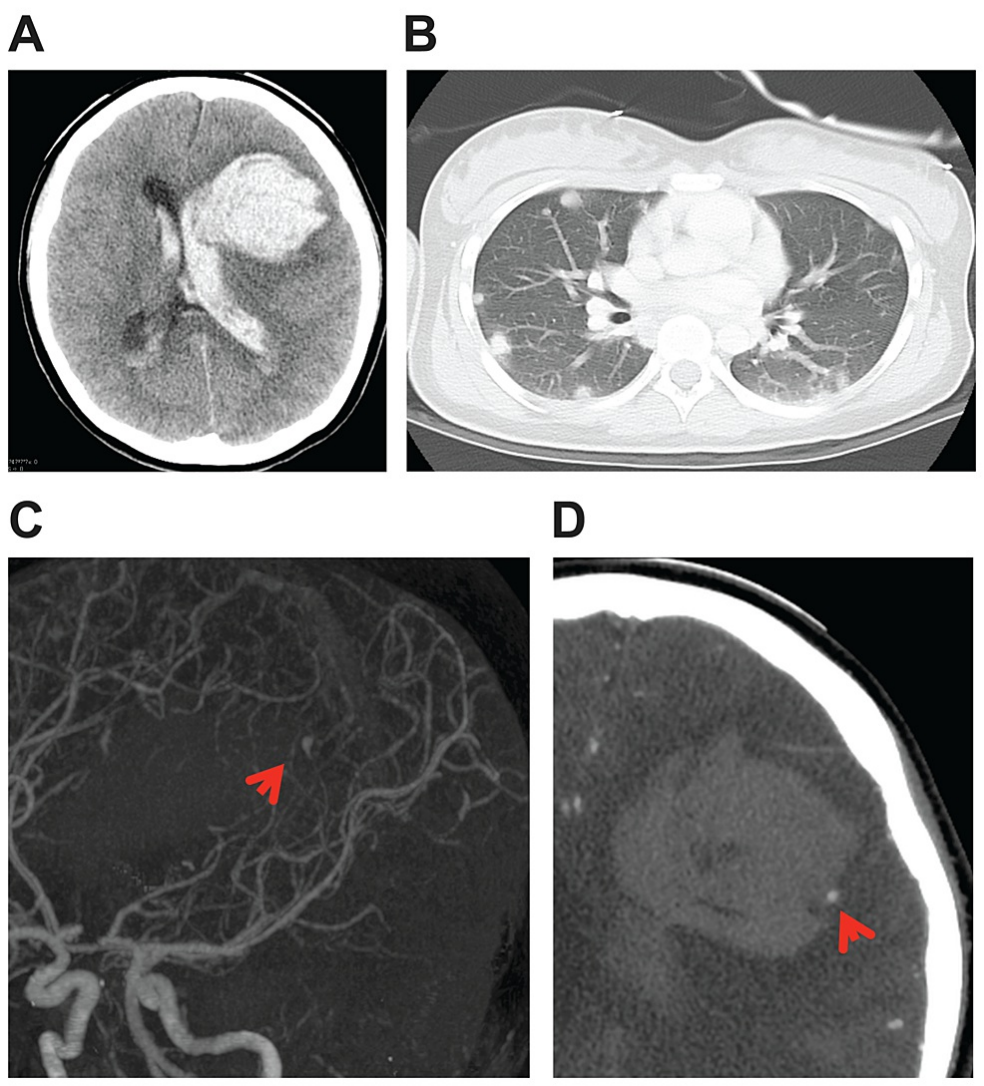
Images obtained in our hospital on admission (A) Head CT scan showed cerebral hemorrhage with ventricular puncture. (B) Chest CT scan showed multiple mass lesions in the lung field. (C, D) Three-dimensional computed tomography angiography revealed the presence of an abnormal vessel, which is suspected as an aneurysm, around the hematoma (arrow).
